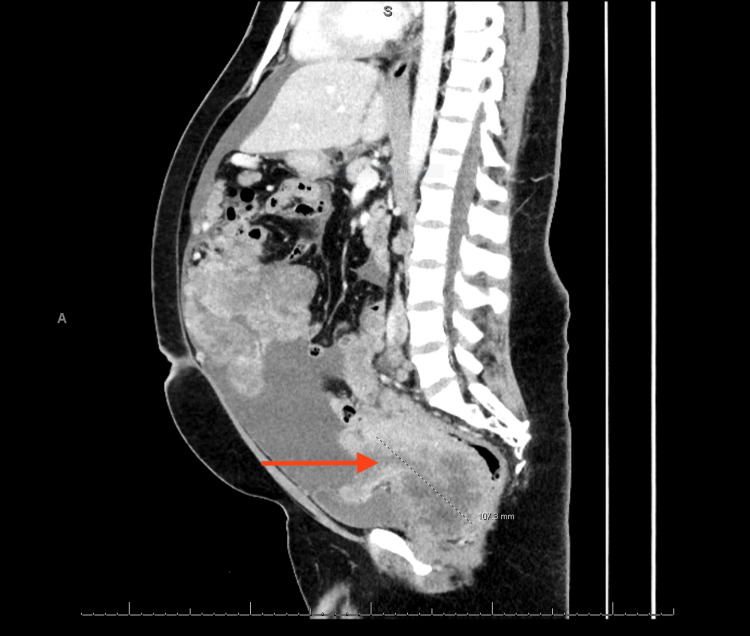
MRI of the abdomen (sagittal cut). The image displays a sagittal abdominal MRI. The red arrow indicates the tumor.
